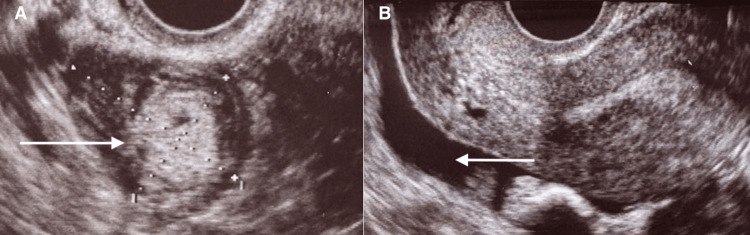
Ultrasound view before surgical exploration 1A: a right-side adnexal mass suggestive of ectopic pregnancy; 1B: sagittal view showing fluid accumulation in the pelvic cavity
